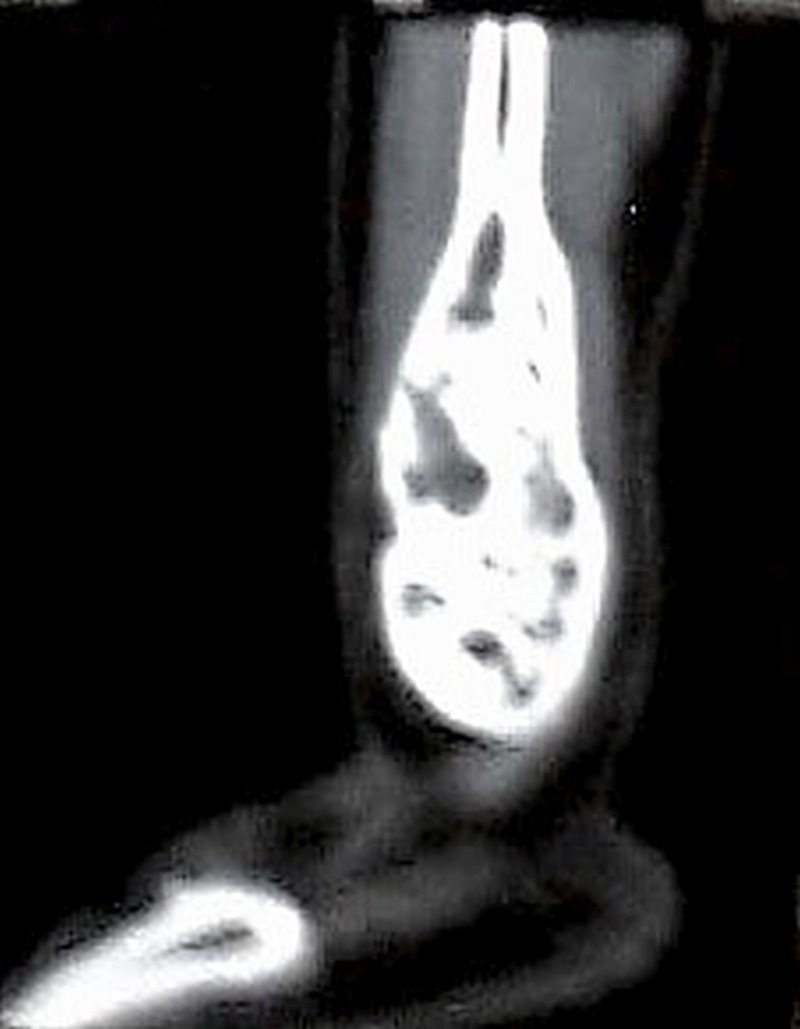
Computed tomography (CT scan)- Sagittal view showing large lesion in distal end of fibula
